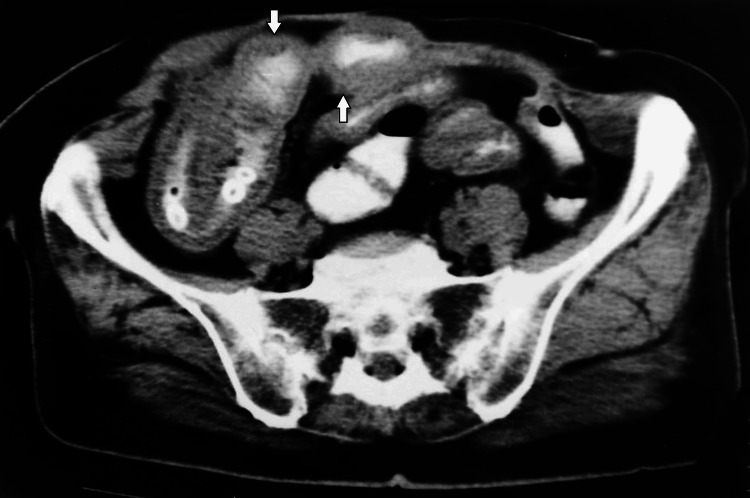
Abdominal computed tomography showing several intraluminal radiopaque foreign bodies in the terminal ileum and thickening and dilatation of the small bowel (arrows).
